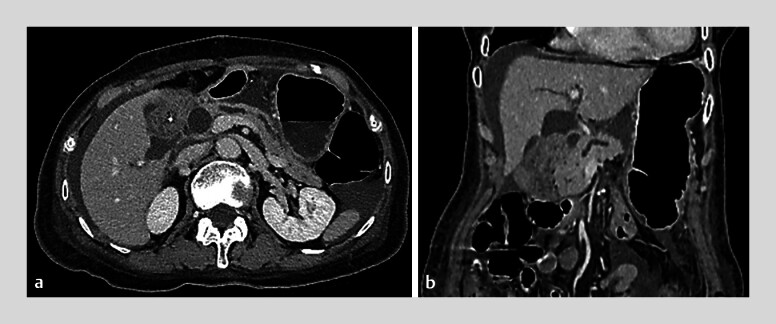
Computed tomography revealed ascites between the stomach and pancreas. The main pancreatic duct was also dilated due to obstruction caused by the ampullary carcinoma.
